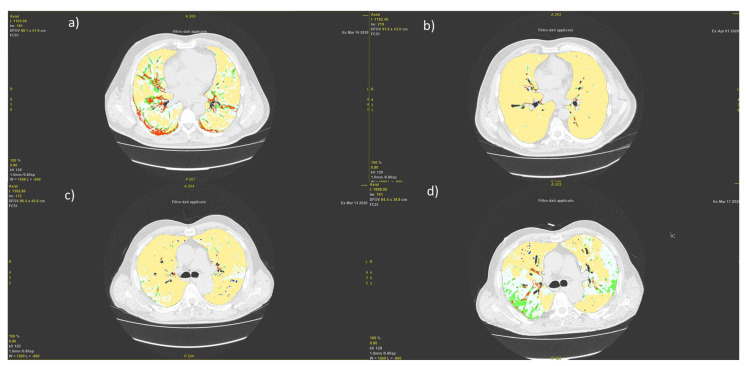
Representative cases of a patient with a CT panel improved and then discharged (a) and (b) (at baseline and follow-up CT), and of a case of a patient with a CT panel worsened and then died (c) and (d) (at baseline and follow -up CT).
